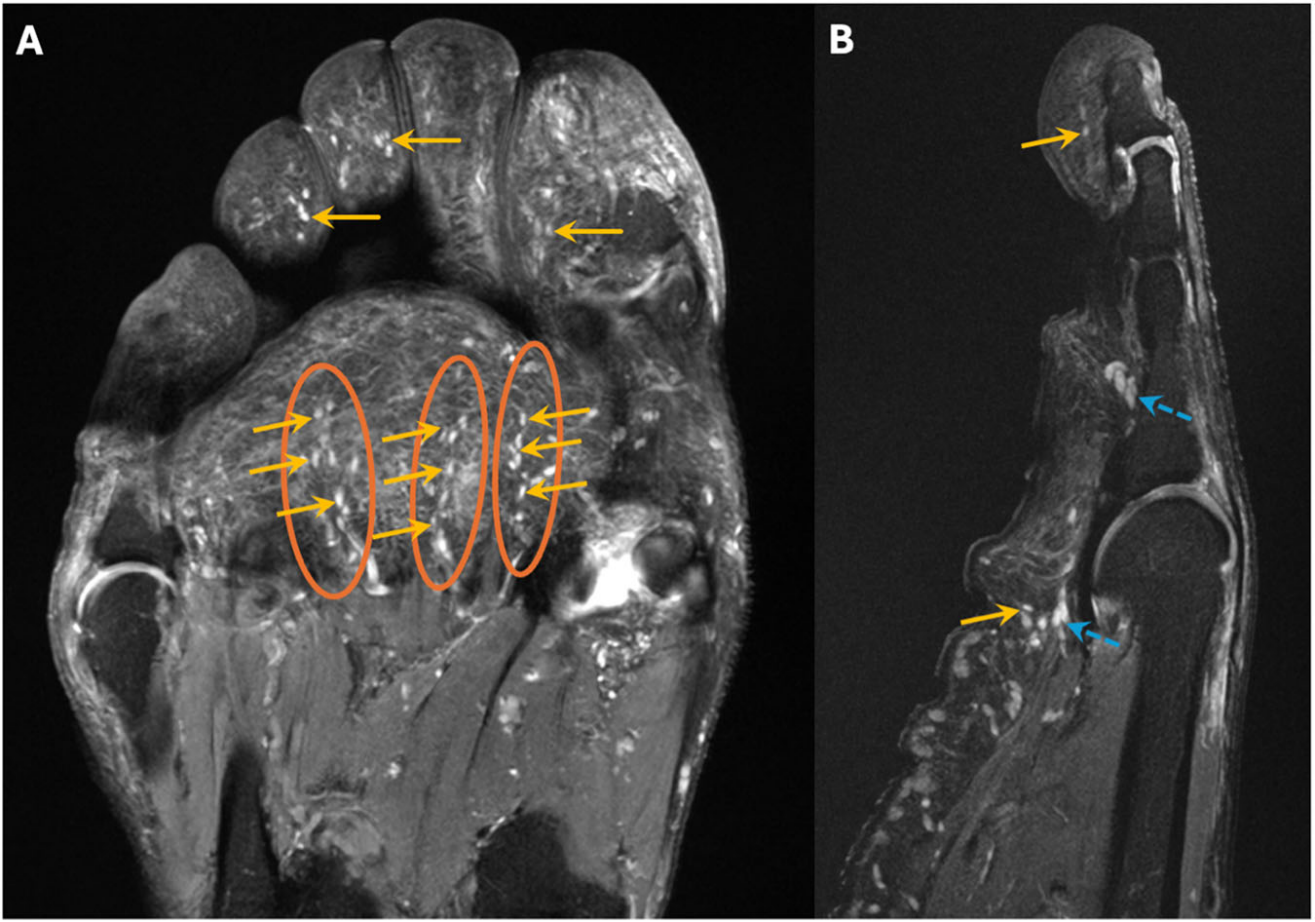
Normal MR-anatomy of Pacinian corpuscles (PC) of a healthy subject on forefoot MRI. Coronal (A) and sagittal (at the level of the fourth digit) (B) proton-density (PD)-weighted fat-saturated (fs) images of the right forefoot of a 60-year-old healthy male volunteer demonstrate numerous homogenously hyperintense ovoid nodules in the plantar subcutaneous fat (yellow arrows, A, B) and along the deep muscle fascia (dashed blue arrows, B), which are identified as PC. In the areas with the most PC, these are predominantly arranged in characteristic “chain-like” patterns, as best seen plantar to the metatarsophalangeal joints and proximal phalanges (circles, A)
